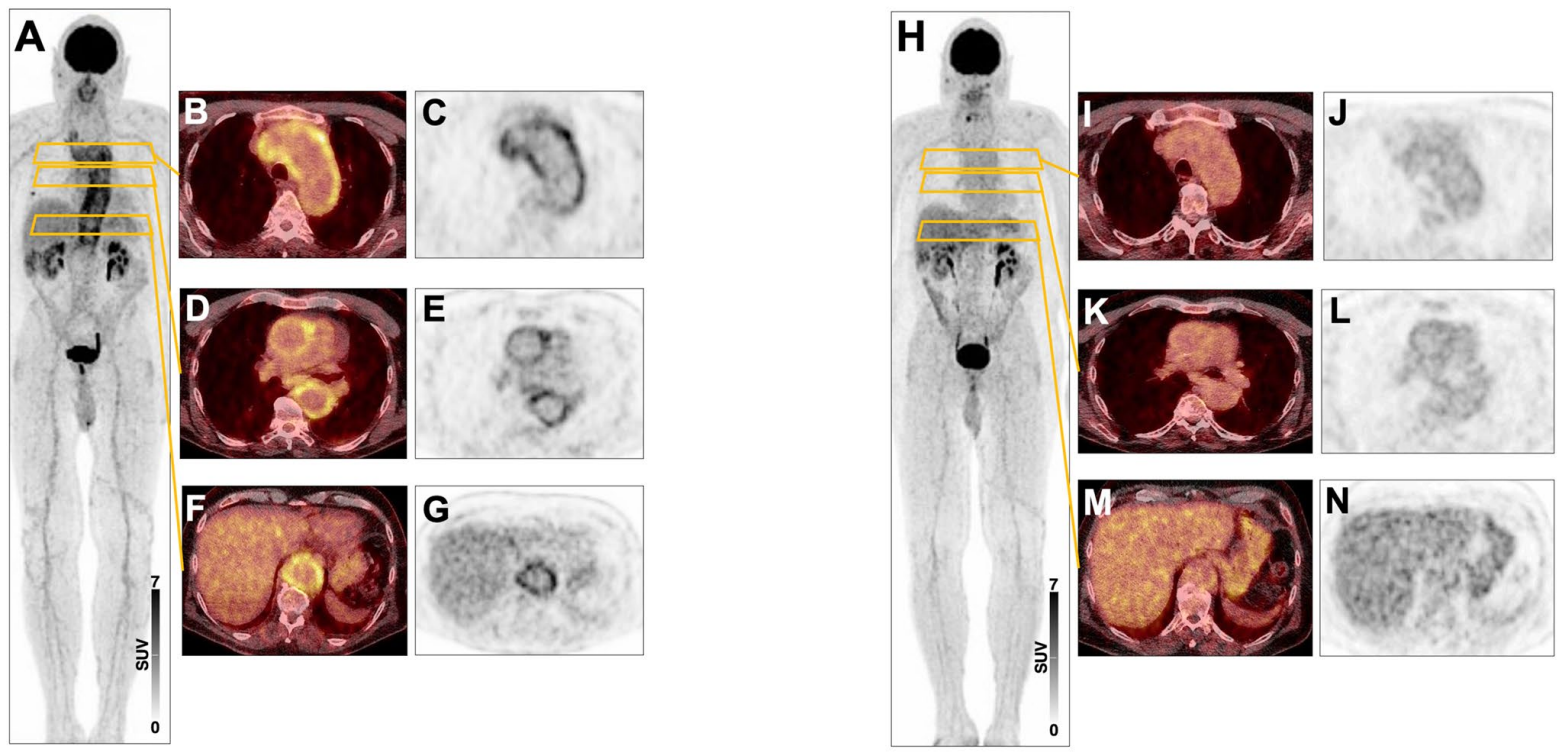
(A) 08/17 Maximum-intensity projection (MIP) ^18^F-FDG PET image showing active large vessel vasculitis in a 3-year-old male patient. (B–G) Transversal fused PET/CT and PET images showing high inflammatory arterial wall signal in the aortic arch (B,C) and the thoracic (D,E) and abdominal aorta (F,G). (H–N) 12/2017 Corresponding PET images after treatment showing markedly reduced signal within arterial walls.
